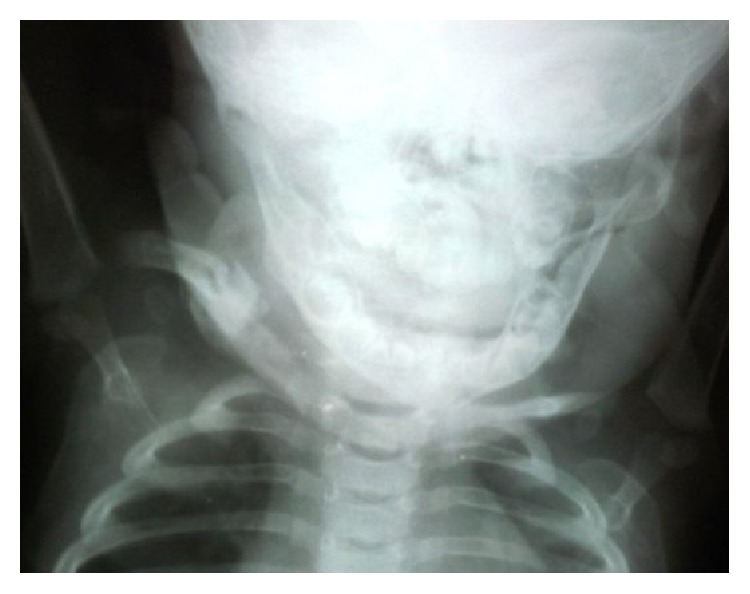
Radiograph six months after operation showing healed osteomyelitis in midshaft of clavicle.
